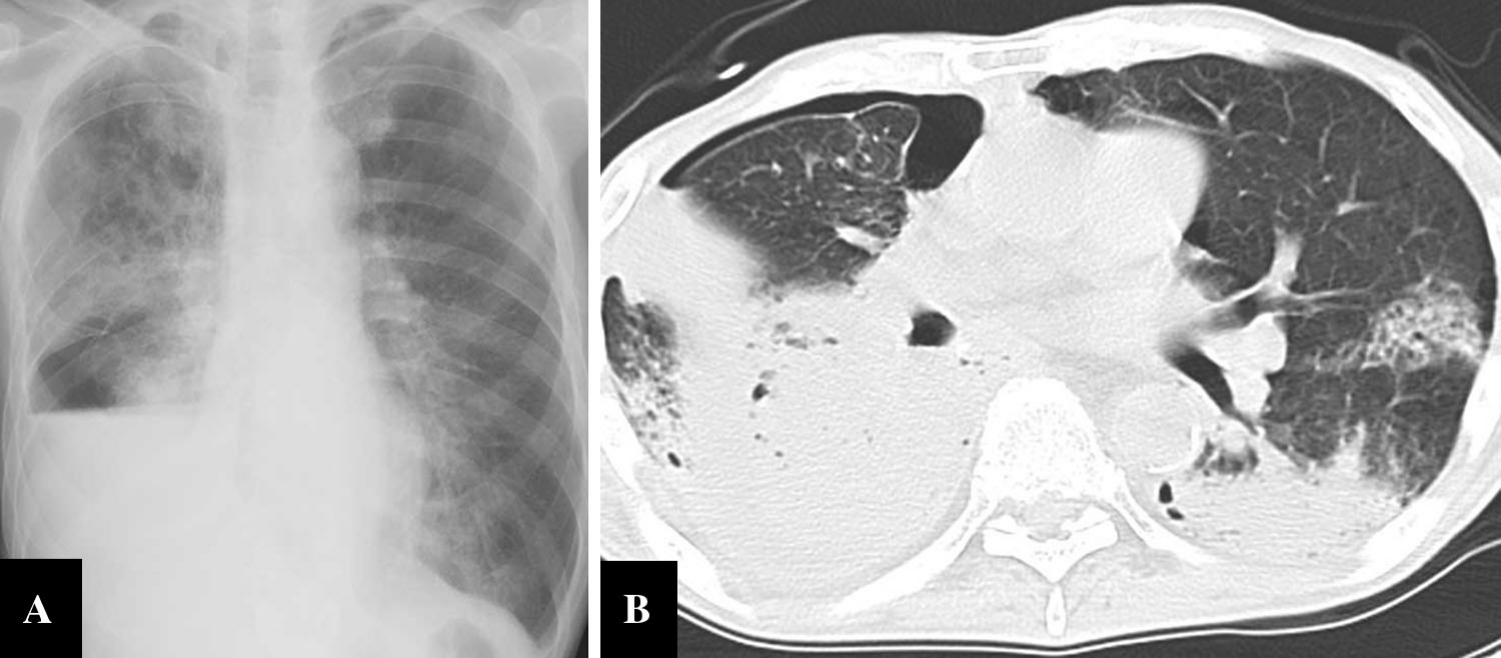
Chest X-ray and CT images upon admission. a Lobar pneumonia of the* right* lung, atelectasis of the *right* inferior lobe and *right* pneumothorax. b Bilateral atelectasis and pneumonia, and* right* pneumothorax
